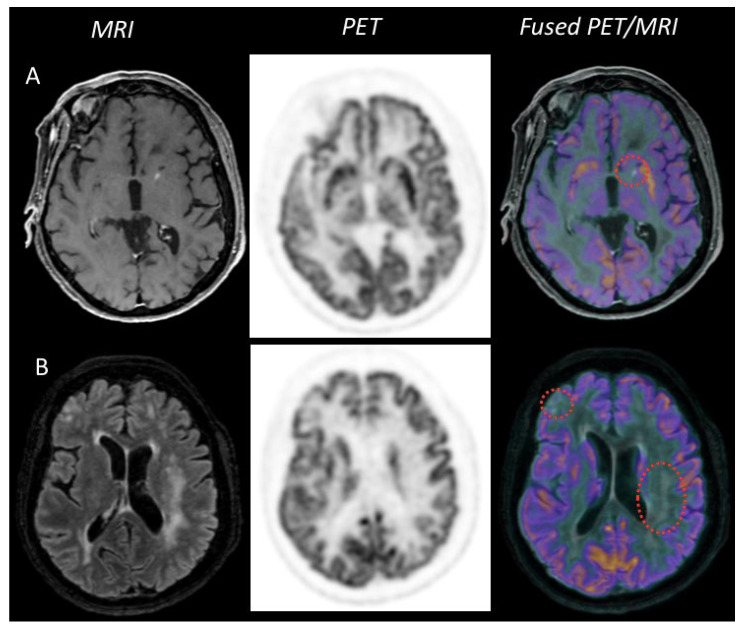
Example case of PET-MRI images of an intravascular lymphoma. Gadolinium-weighted T1+ imaging (A) showed a small enhanced punctiform lesion in the medial part of the left striatum, with no metabolic uptake on ^18^F-FDG PET images. The cortical and subcortical lesions in FLAIR hypersignal (B) did not show uptake with ^18^F-FDG either.
